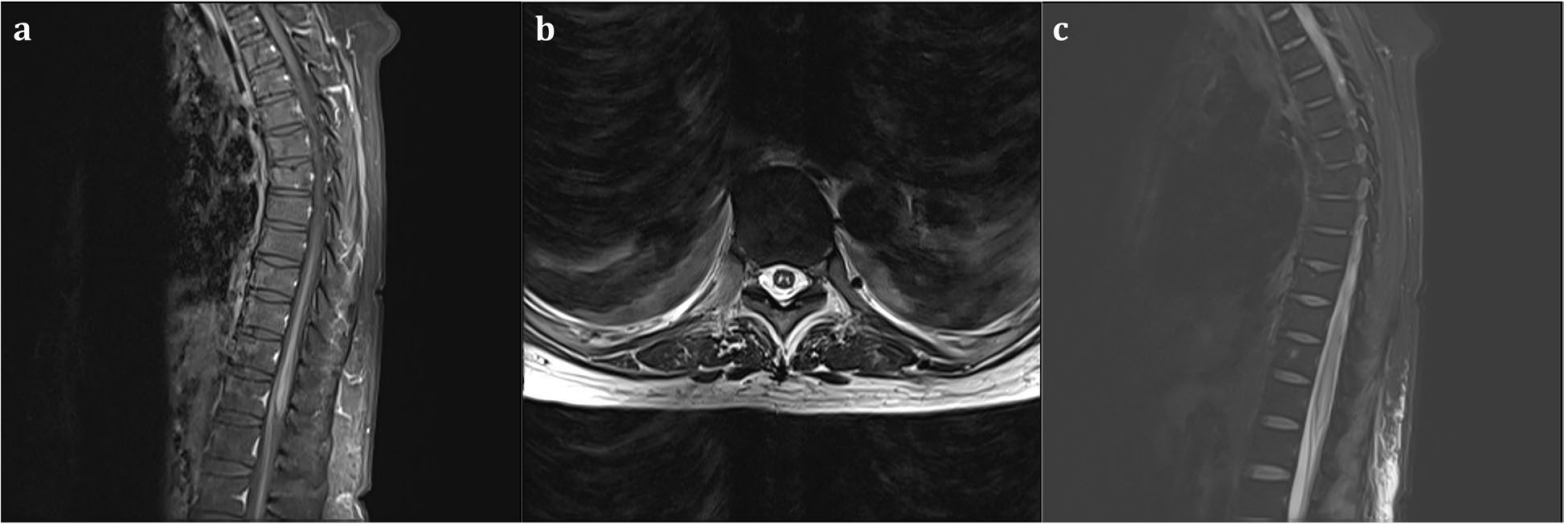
Thoracic spinal cord MRI. Sagittal T1-weighted MRI shows signal enhancement in the gray matter of the spinal cord (a); Axial T2-weighted MRI shows increased signal intensity in the gray matter segment of the spinal cord (b); Sagittal fat-suppressed sequence MRI shows enhanced signals of spinal cord swelling (c)
